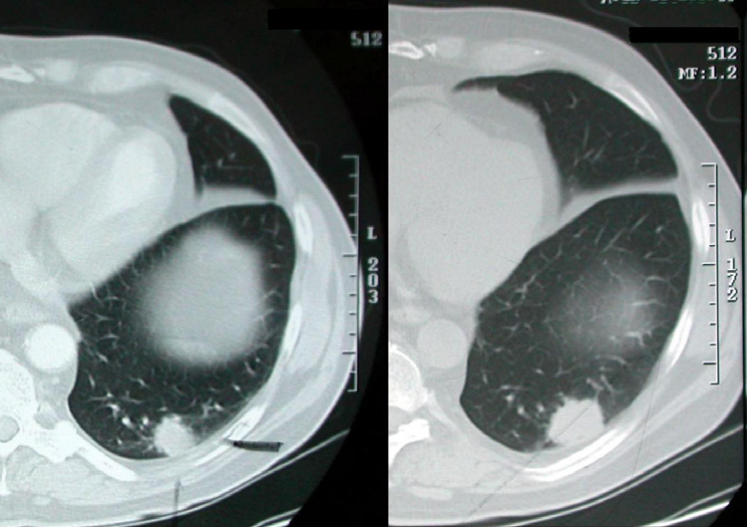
Chest CT scan showing a 1.7 cm left lower lobe nodule (*left*), as well as, a three months follow up Chest CT scan demonstrating enlargement of the left lower lobe nodule from 1.7 cm to 3 cm (*right*).
